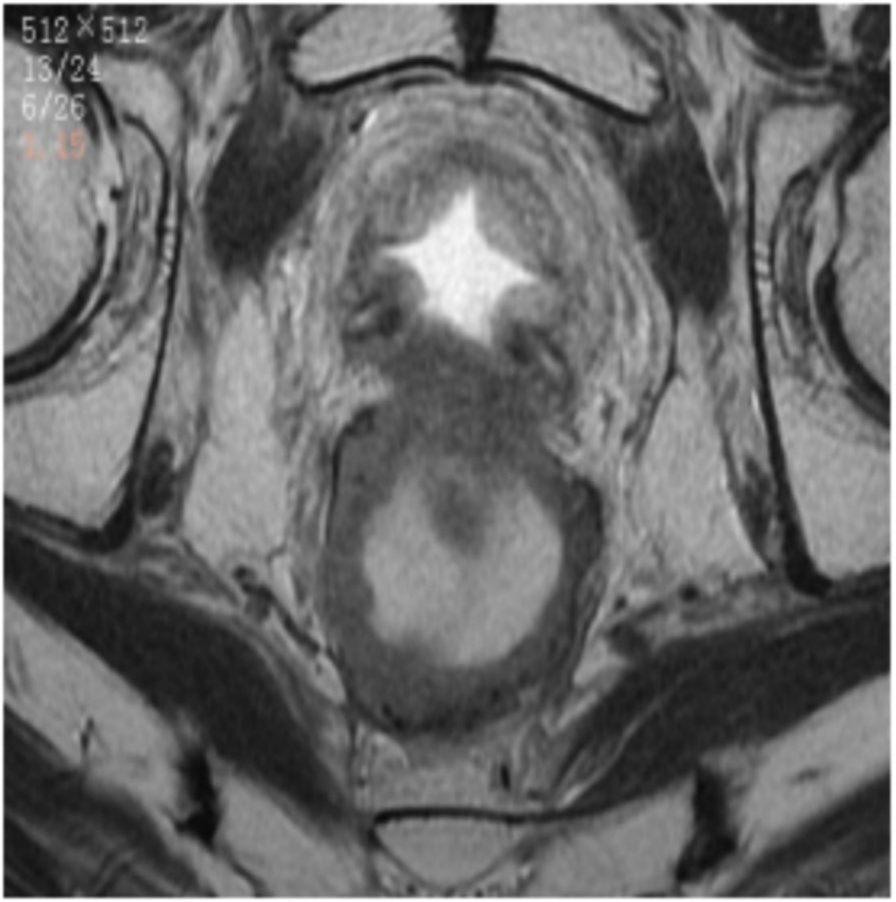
MRI showing irregular circumferential thickening of the rectum wall at the surgical site
